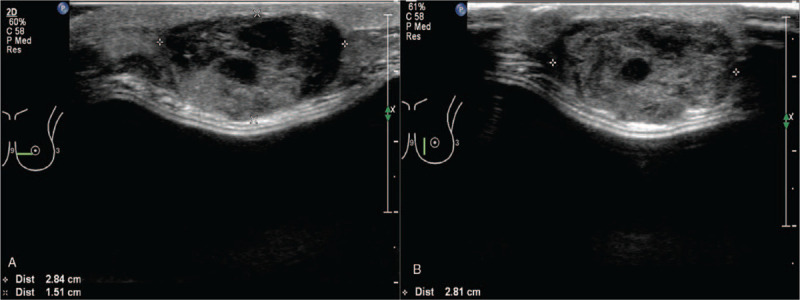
Transverse (A) and longitudinal (B) ultrasonography of the lower inner region of the left breast mass demonstrated a well-defined hypoechoic lesion accompanying anechoic appearance.
